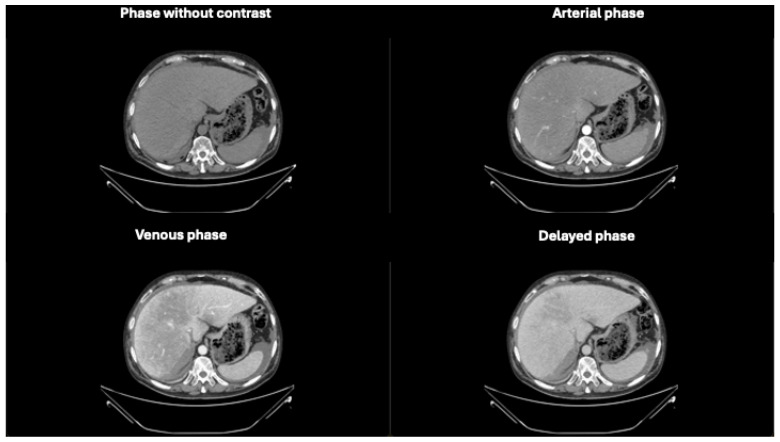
Axial phases without and with contrast CT images demonstrating hepatomegaly with a liver length of approximately 25 cm. The liver parenchyma displays a finely and diffusely heterogeneous density.
